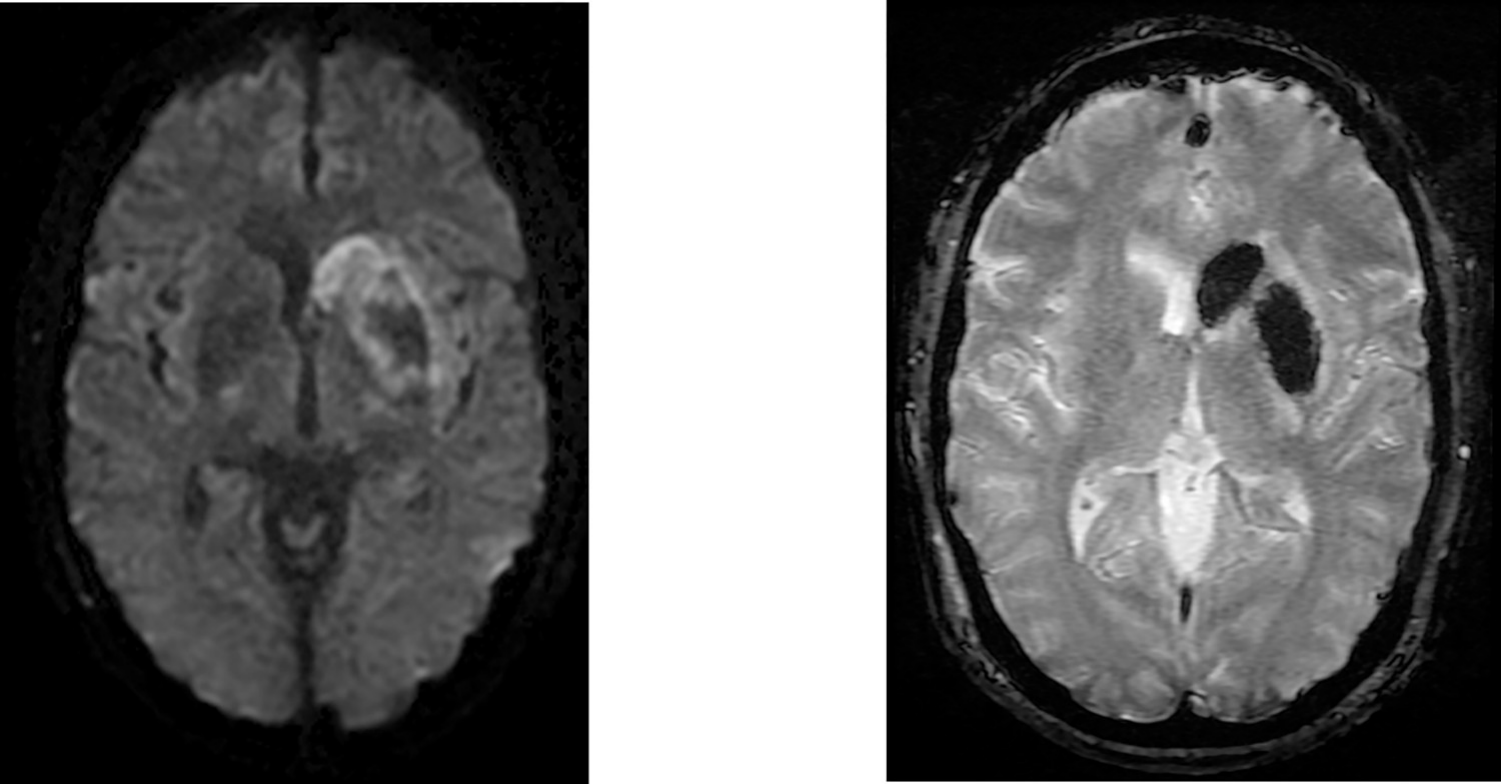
Imaging findings in a 54 y.o. female COVID-19 patient with an endometrial carcinoma who was not receiving ventilatory support. An acute infarct in the left basal ganglia is shown in the DWI image on the left. The heterogeneous signal pattern through the infarct bed is explained in part by the axial gradient echo image on the right, which shows susceptibility through much of the same territory implicating hemorrhage conversion.
